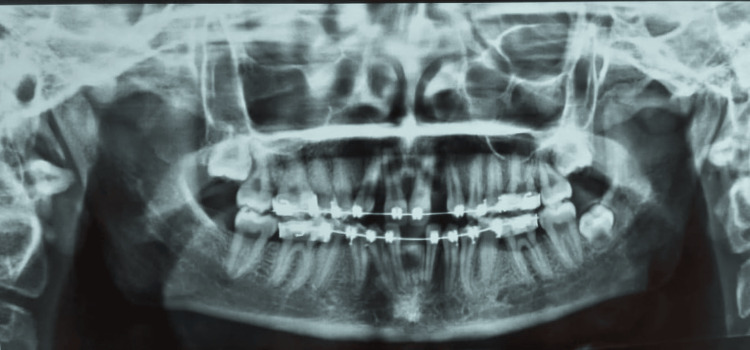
OPG image OPG: orthopantomogram
